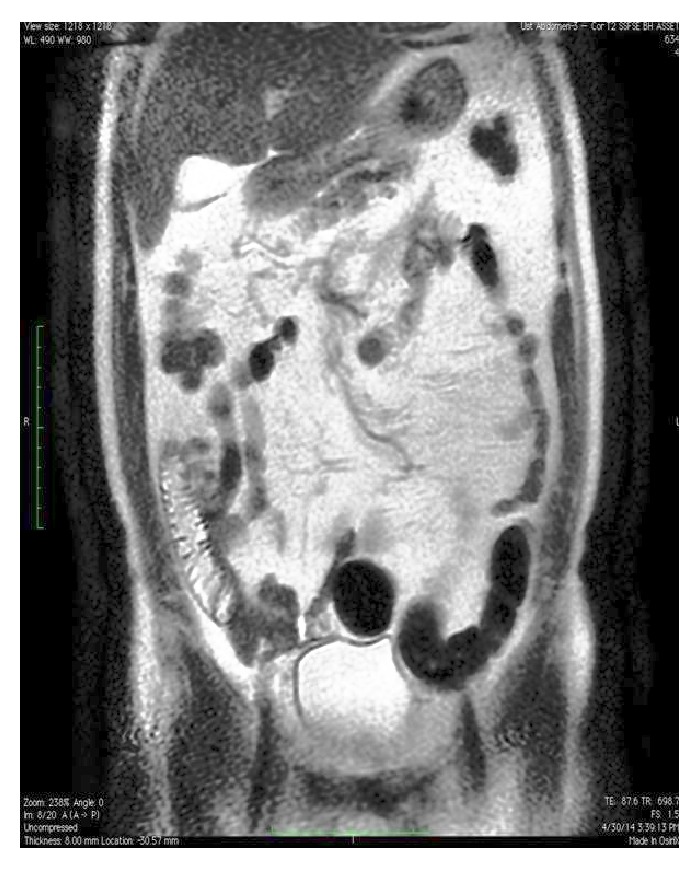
Coronal T2 weighted MR imaging with diffuse abdominal lipomatosis of the father of index case.
